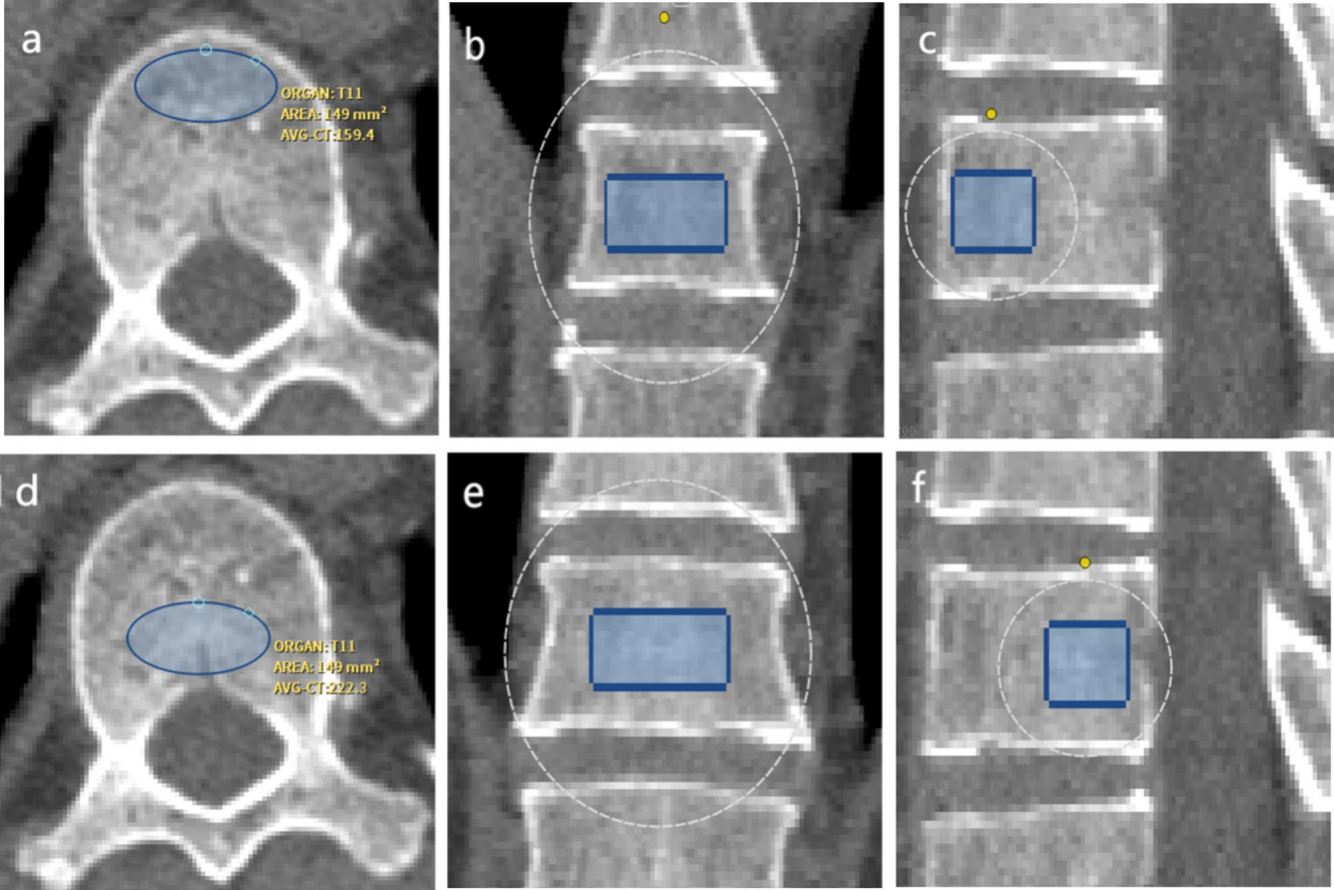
CT images showing bone density measurement assessments of the anterior and posterior vertebral body in axial, coronal, and sagittal planes. Figures (a–c) present the axial, coronal, and sagittal measurements of bone density in the anterior vertebral body. Figures (d–f) display the axial, coronal, and sagittal measurements of bone density in the posterior vertebral body.
